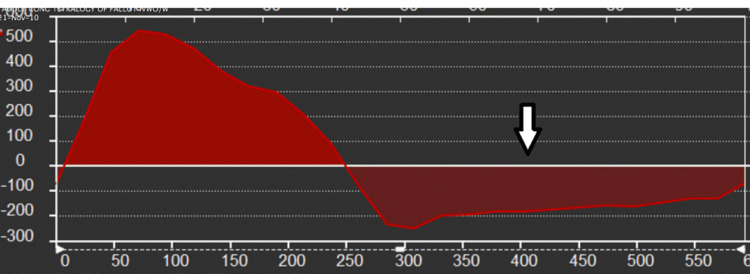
Cardiac Magnetic Resonance Imaging. Aortic flow analysis shows a very large aortic regurgitant fraction of 71%. In the absence of significant visual aortic valve insufficiency, this reflects large left-to-right flow across the SOVAR. Arrows highlight the portion of the aortic flow curve below the line, which represents the regurgitant flow. The flow above the line is the forward flow, and the ratio between both provides the aortic valve regurgitant fraction.
